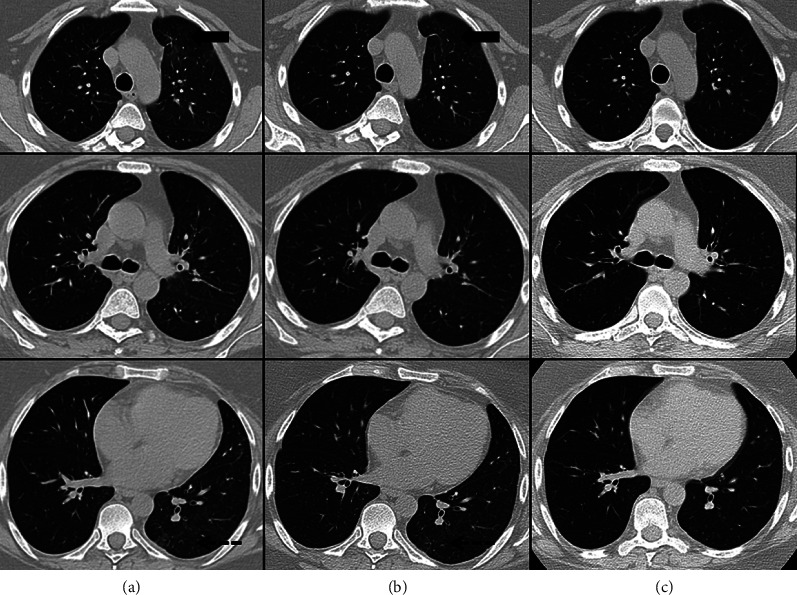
Three-(a), and six-month follow-up (b) chest HRCT images: stable ground glass opacity (GGO) and interlobular interstitial thickening, mainly in the peripheral-subpleural areas of the left lung; some fibrotic-disventilative stripes are unchanged at the anterior segment of the upper lobe (black arrow) and at the apical segment of the lower lobe, at the dorsal site (pointed arrow). Twenty-six-month follow-up (c) chest HRCT images: almost complete resolution of interstitial lung disease, and only a thin fibrotic-disventilative stripe remains at the anterior segment of the left upper lobe.
